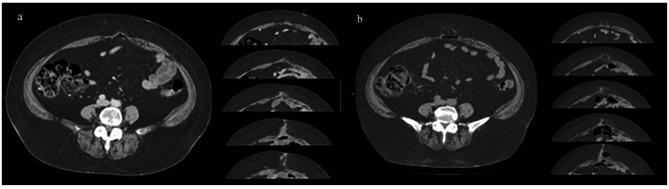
Example of CT images of diagnosed IH occurrence. a) Computed tomography (CT) images at 6 months postoperatively. The black line indicates the fascial defect. The length of the horizontal axis was 1.7 cm (minor axis). Six slices of the CT images showed fascial defects; the length of the vertical axis was 3.0 cm (the major axis). The incisional hernia (IH) size was calculated as the ellipse area at 4.0 cm^2^b) CT images were taken 1 year postoperatively. The black line indicates the fascial defect. The length of the horizontal axis was 3.4 cm (minor axis). Fifteen slices of the CT images showed fascial defects; the length of the vertical axis was 7.5 cm (the major axis). The size of the IH was calculated as the elliptical area at 20.0 cm^2^
